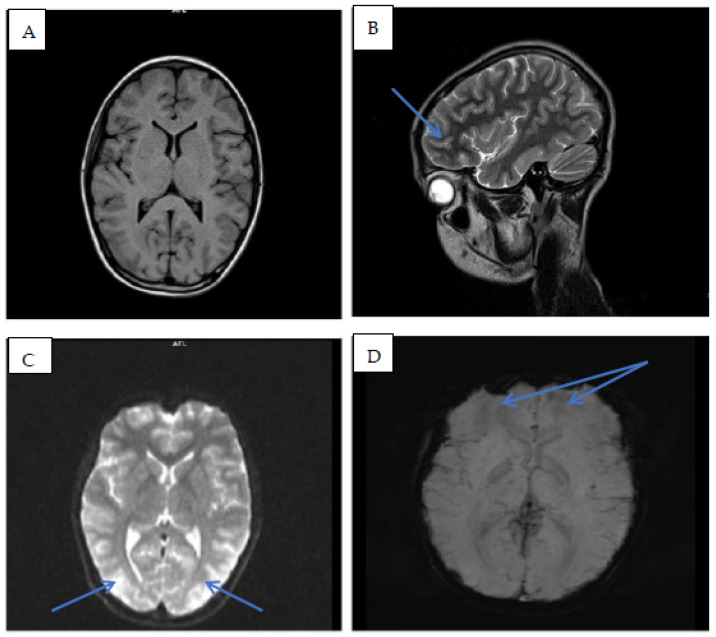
Magnetic resonance imaging with contrast. Legend: Brain MRI performed on the second day after onset of symptoms detected increased signal on Flair- and T2-weighted imaging in the subcortical and cortical regions of the parietal-occipital areas (axial FLAIR images (A,C,D)). Also, the superior frontal area showed the same changes (sagittal view (B)), pointed out by the blue arrows. However, normal diffusion without hemorrhages were identified (A). The diagnosis of PRES was made after imaging was performed.
